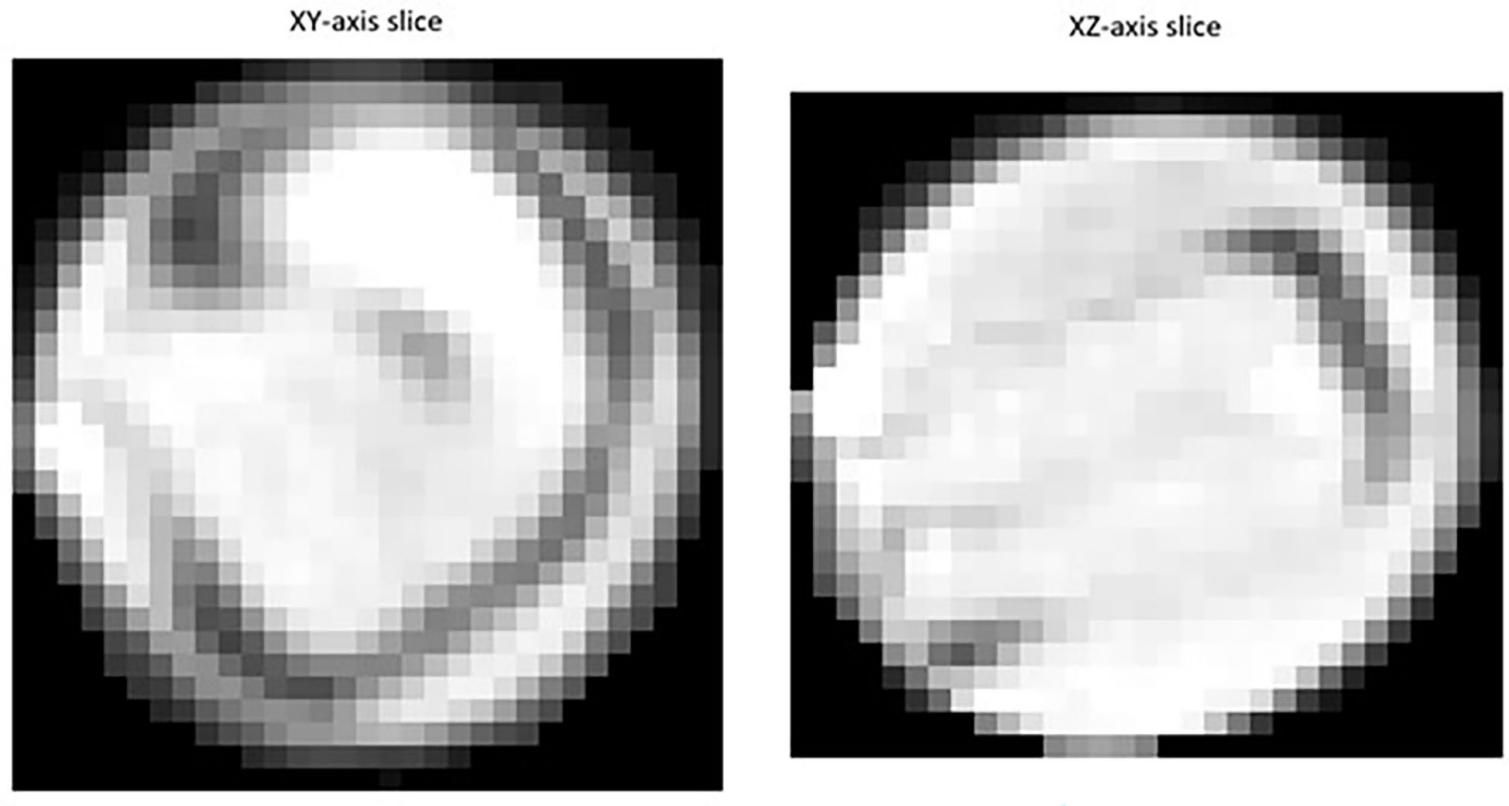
Example of a 2D slice images extracted for individual *Brassica napus* seeds detected and segmented with a fine-tuned StarDist-3D algorithm from 3D micro-computed tomography.
